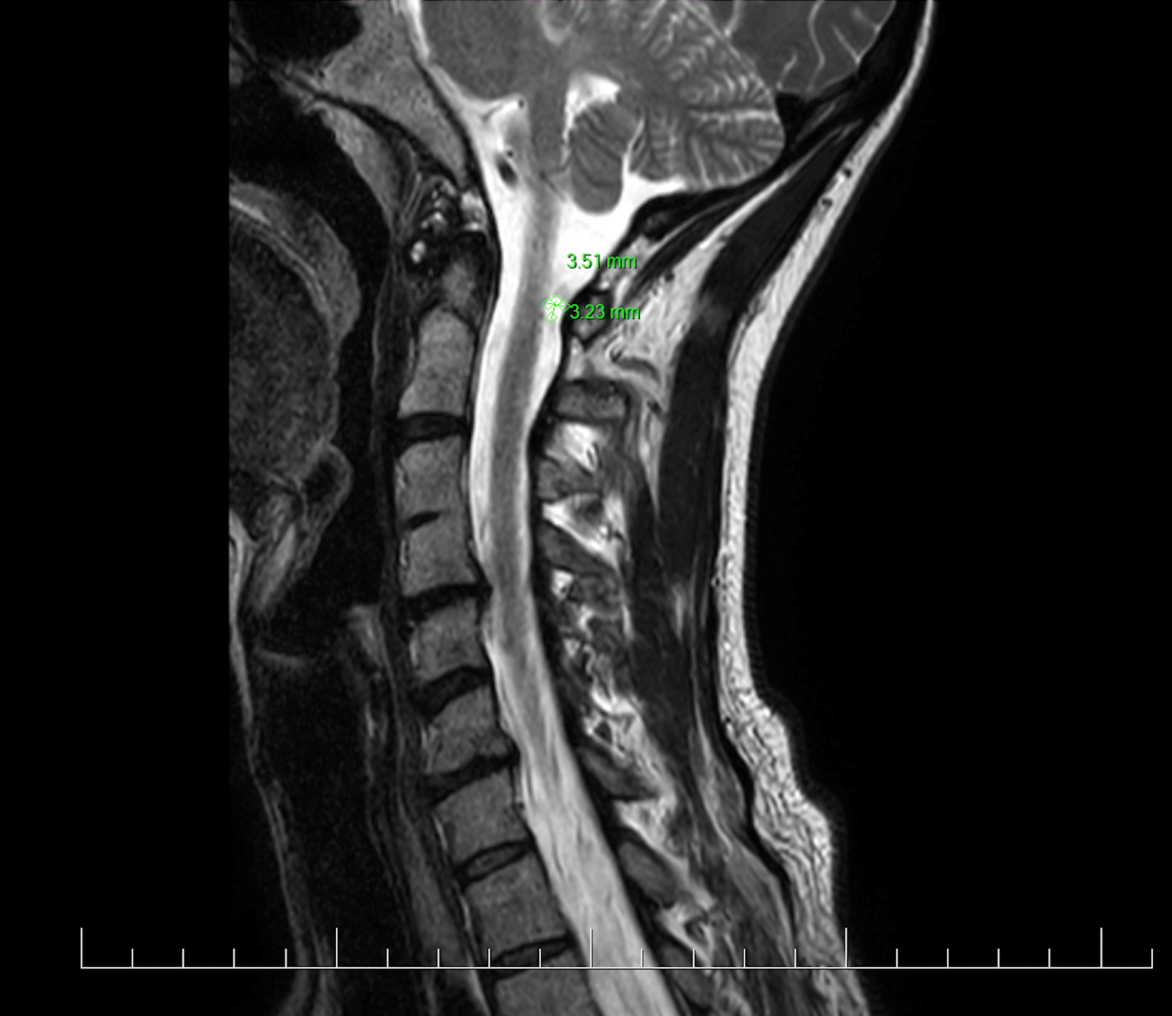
MRI of cervical spine nine months after augmentation MRI of the cervical spine showing a suspected meningioma in the spinal canal at C1 (green markings).
